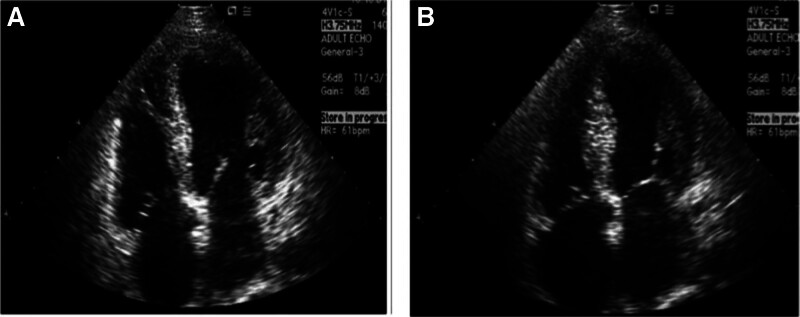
Initial echocardiography showing apical ballooning at diastole (A) and at systole (B) of apical 4-chamber view.
